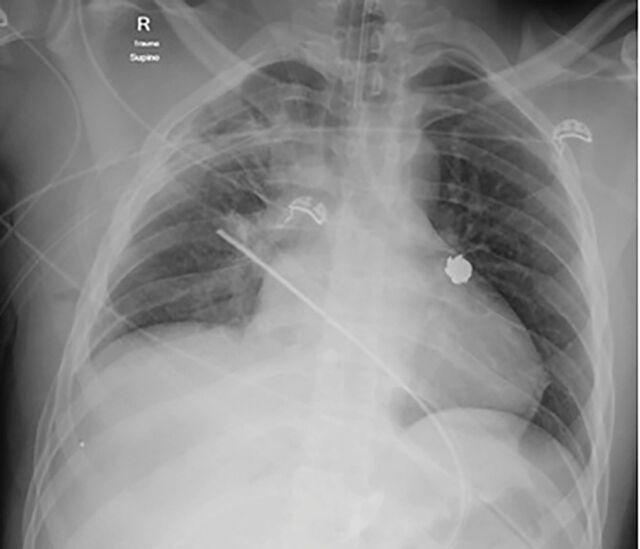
Initial chest x-ray showed a fluid-filled right chest with a bullet projecting over the left lung hilum.
